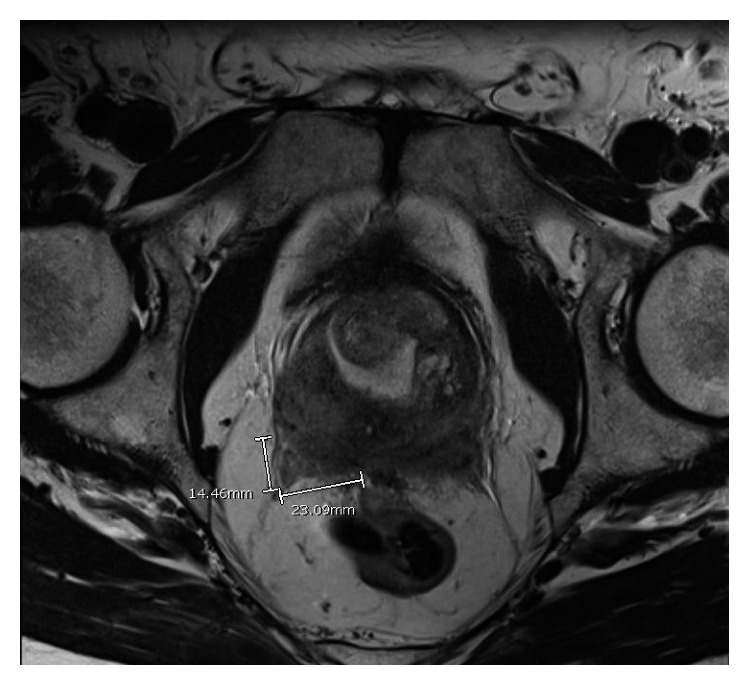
Pelvic MRI at diagnosis (axial view).
